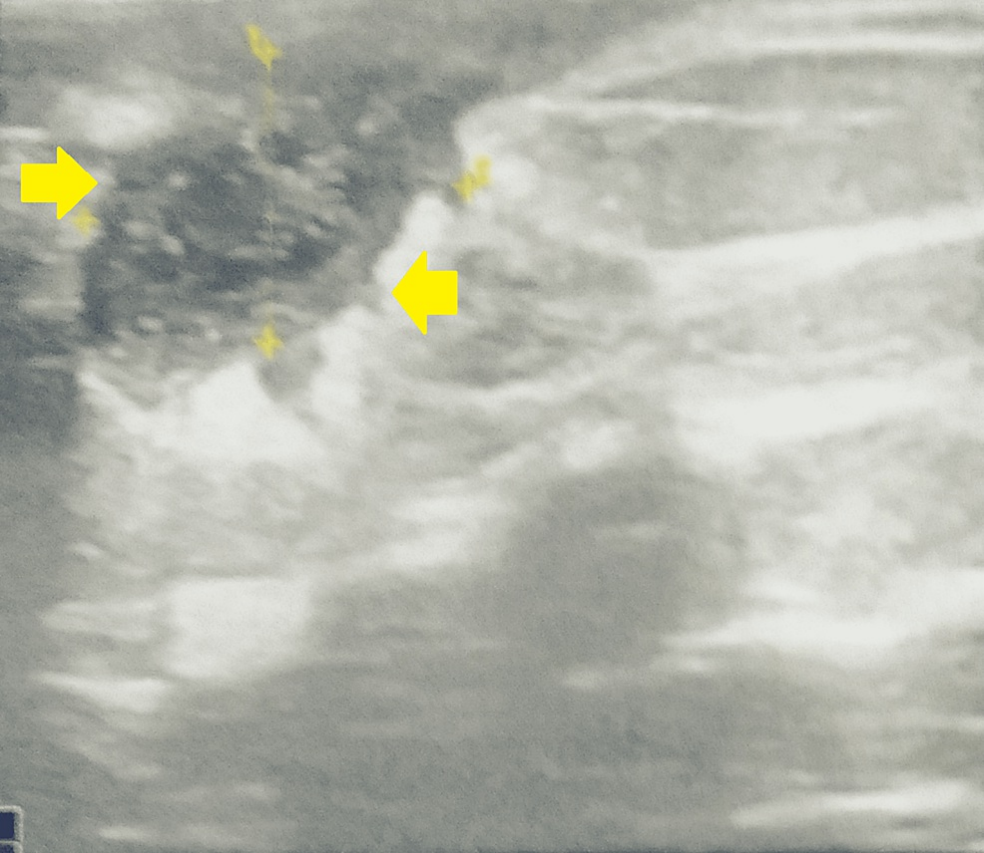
An ultrasound of the breast showing a heteroechoic collection in the right breast
